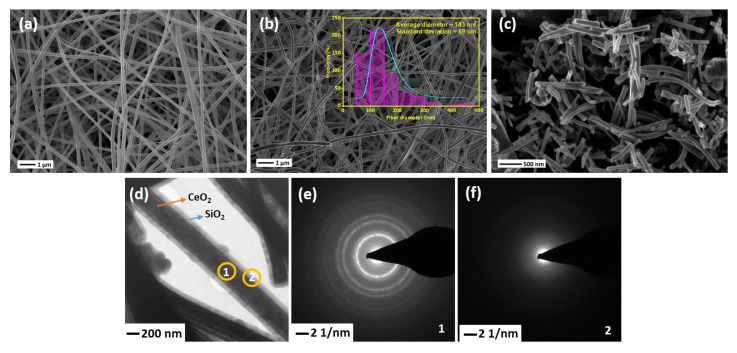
SEM images of (a) as-spun Ce(NO_3_)_3_·6H_2_O/PVP NFs, (b) CeO_2_ NFs after calcination at 600 °C, Insert shows the size distribution plots of fibers diameter, (c) CeO_2_ NF after 10 min ultrasonication, (d) TEM image of CeSi, (e,f) SAED of CeSi, core (1) and sheath (2).
